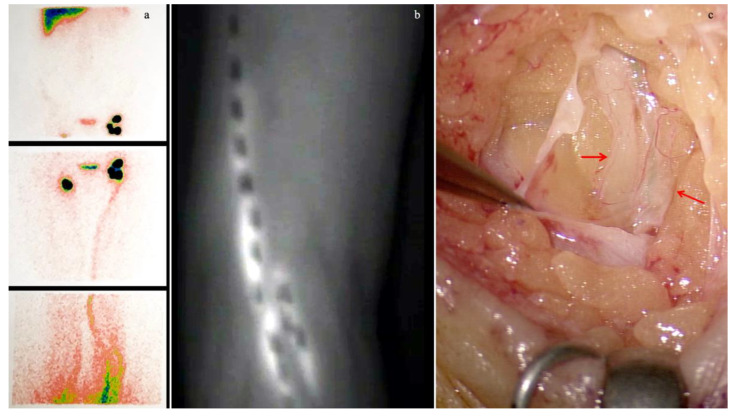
This figure shows the instrumental and intra-operative features typical of early-stage lower limb lymphedema. (a) Preoperative lymphoscintigraphy aimed at the superficial lymphatic system of a patient affected by early-stage lymphedema of the left lower limb secondary to Pelvic Lymphadenectomy (PL) for gynecological cancer. The lower part of the picture shows the leg functioning ectasic pathways with some extravasation in the left leg. In the middle figure, functioning vessels are present in the thigh. The image also reveals functioning inguinal lymph nodes that uptake the radiotracer. In the upper part of the picture, no pelvic lymph nodes are present; (b) Indocyanine Green Lymphography (ICG-L) of the same patient shows splash pattern, typical of ectasic lymphatic vessels; (c) intra-operative picture of the same patient during surgery shows ectasic lymphatic vessels, indicated by red arrows. In one of the two vessels, green dye is visible inside the lumen.
